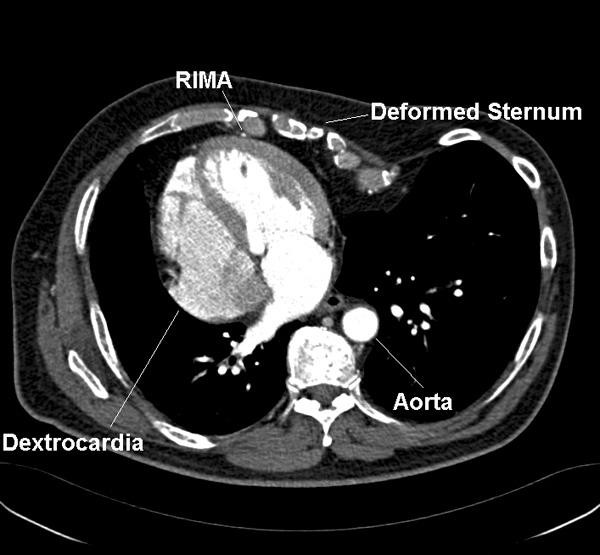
CT scan showing dextrocardia with situs solitus. (RIMA- Right Internal Mammary Artery).
